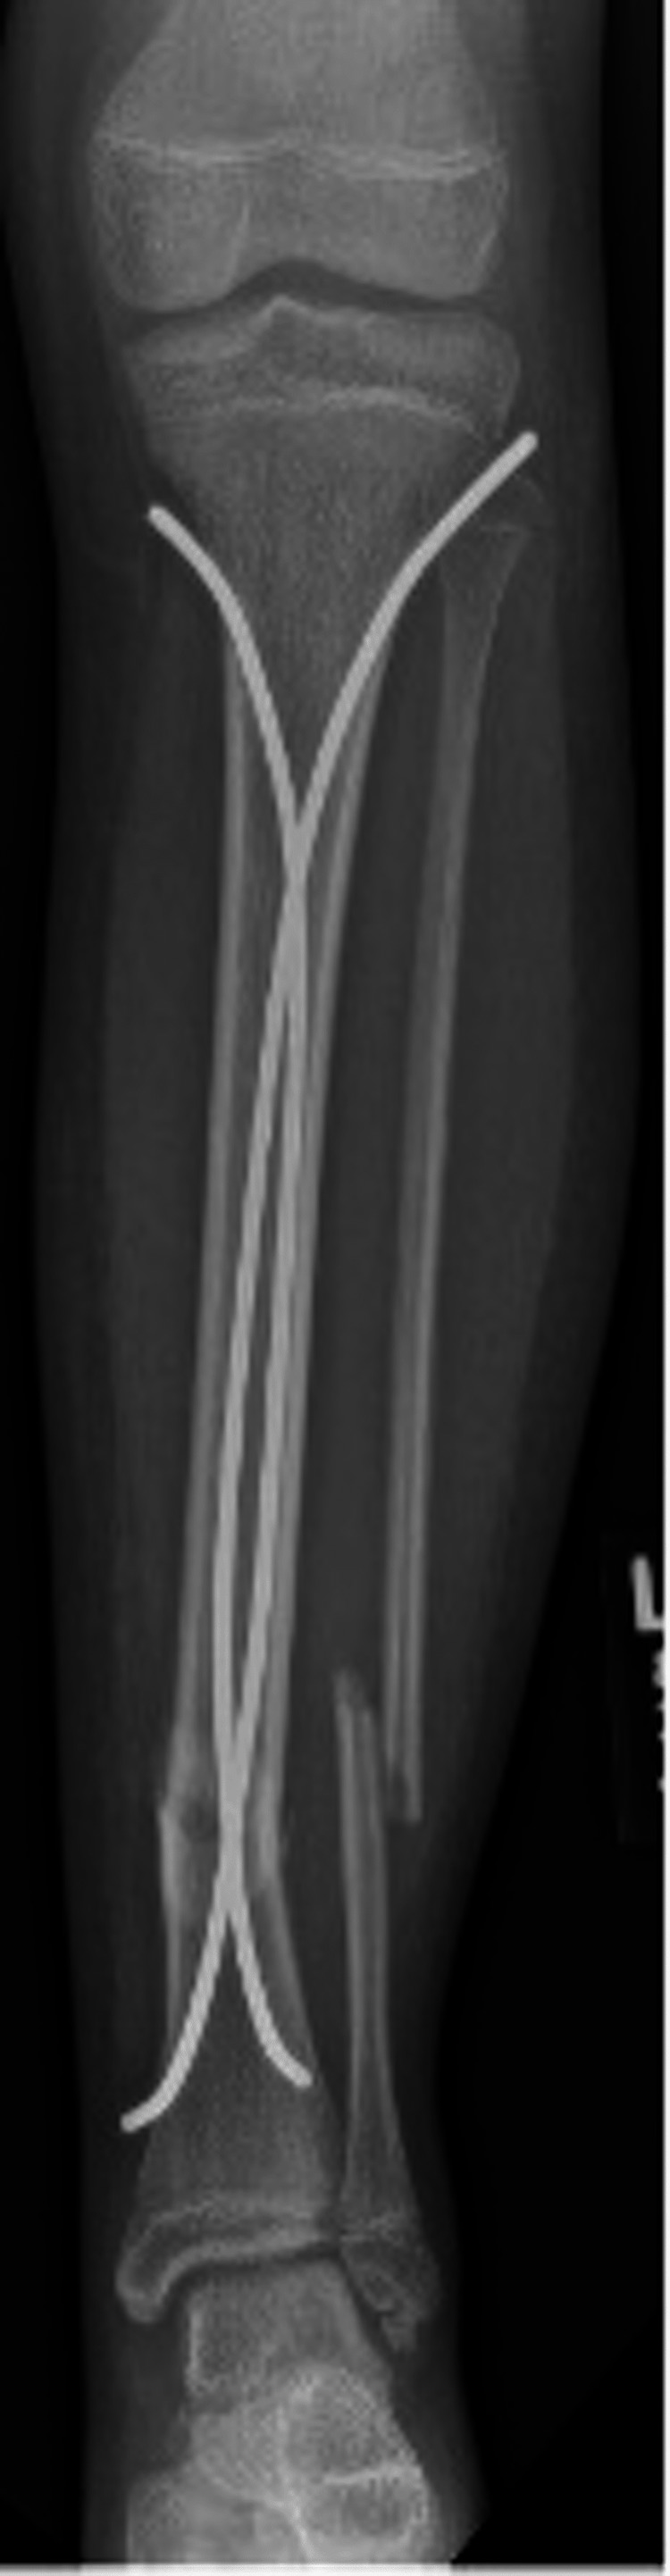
One-month post-operative anterior-posterior tibia-fibula radiographs of flexible nailing of the tibia.
